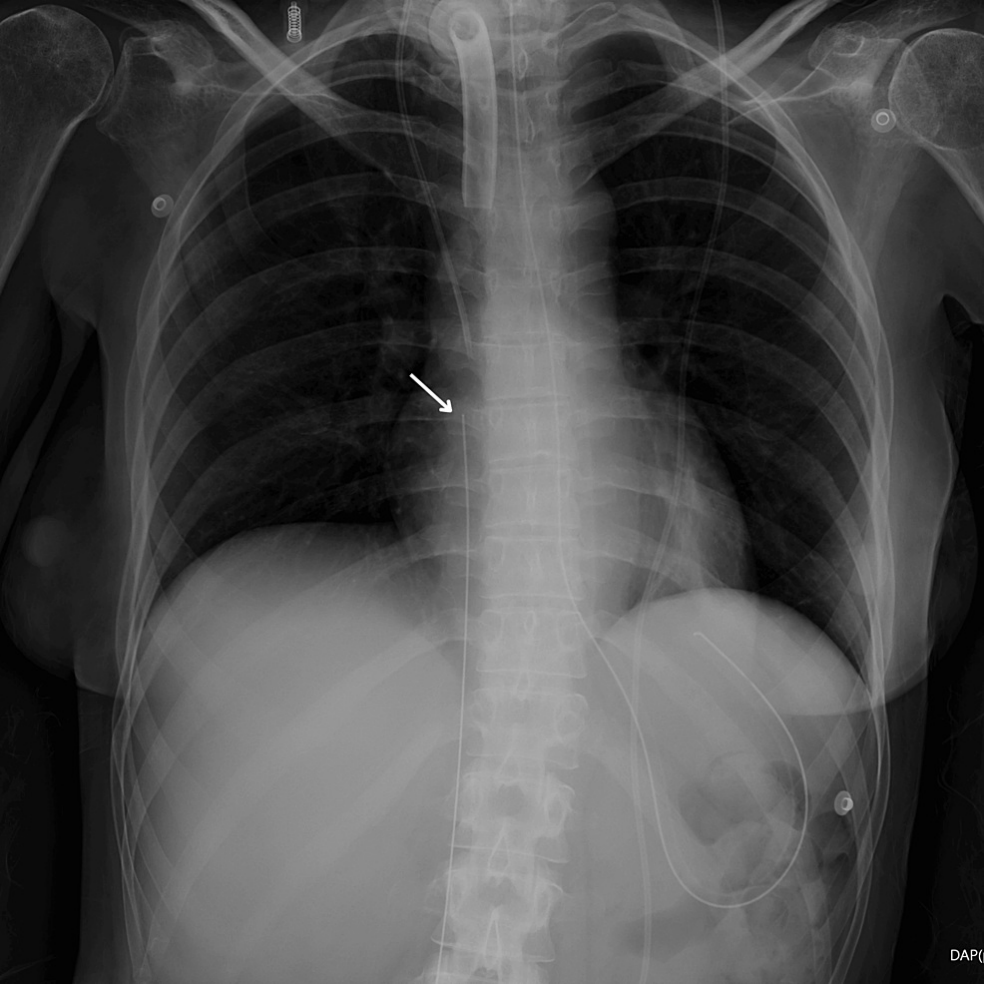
Chest X-ray The proximal part of the guidewire
